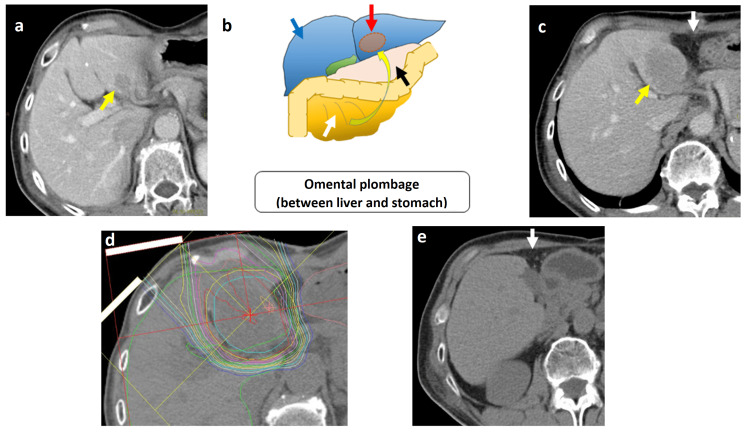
Timeline of proton beam therapy with space-making surgery (omental plombage) for oligorecurrent liver metastasis of esophageal adenocarcinoma. Contrast-enhanced CT showed the lesion in S2 (yellow arrow) (a). The liver metastasis in S2 was very close to adjacent organs including the esophagus and the remaining stomach, and we therefore decided to perform the space-making surgery using the remaining omentum after the proximal gastrectomy. An image of space-making surgery using the omental plombage is shown. Blue, white, red, and black arrows indicate the liver, great omentum, tumor, and stomach, respectively (b). After space-making surgery, CT showed that the omentum (white arrow) was located between the liver metastasis in S2 (yellow arrow) and stomach (c). The second PBT for the liver metastasis in the S2 was performed with 72.6 GyRBE in 22 fractions (d). Seven years after the second PBT, a complete response was maintained and the inserted omentum has still remained (e).
